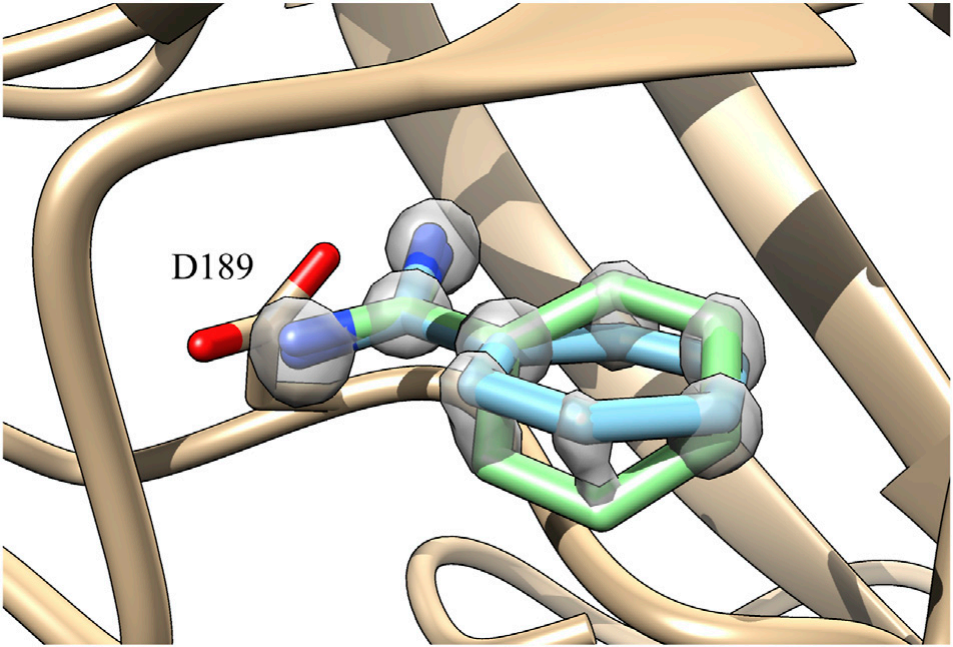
Superimposition with the X-ray electronic density map of the two benzamidine conformations obtained with the *ad hoc* parametrization having the dihedral angle at 0.5 and −0.5 radians shown in green and cyan, respectively. The density map of benzamidine has been represented at a contour level of 2.6 Å and transparency 0.5. The density map of the protein has been undisplayed for clarity reason (Liebschner et al., 2013).
